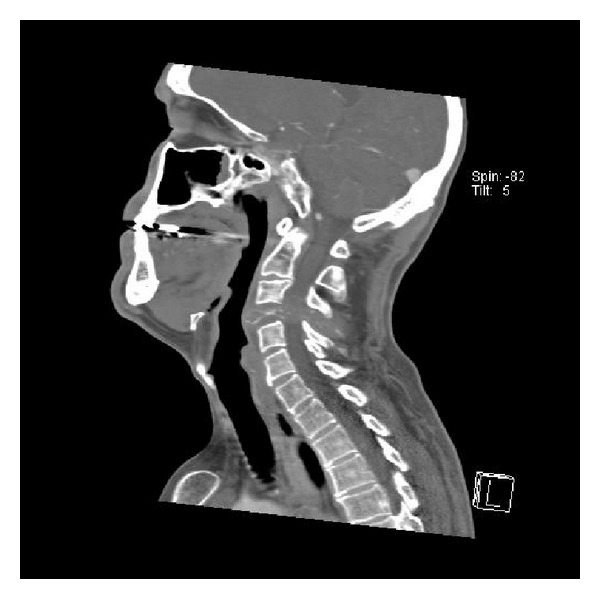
CT of the neck demonstrating erosive metastatic tumor of the C4 vertebral body.
